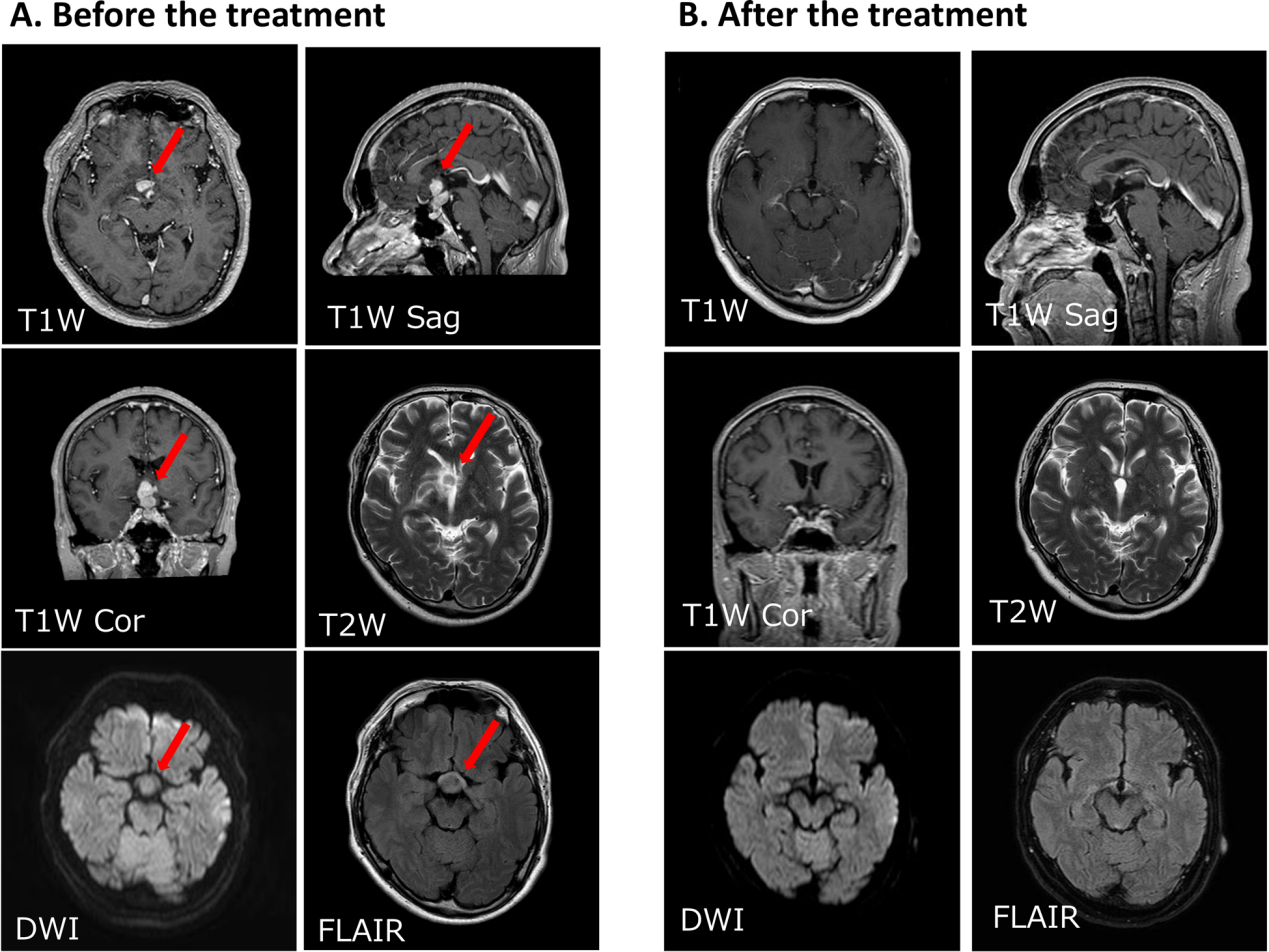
Imaging analysis before and after the treatment of the central nervous system lymphoma. a, b. Gadolinium-enhanced magnetic resonance imaging (MRI) before and after the initial treatment of the patient’s central nervous system lymphoma. A 16 × 12 mm lobulated tumor mass in the hypothalamus that extends to the pituitary stalk and the right temporal lobe was detected as a low intensity mass in T2-weighted imaging, a faint high intensity mass in diffusion-weighted imaging, and fluid attenuated inversion recovery imaging (a). The mass was no longer detected after the treatment (b). T1, T1-weighted imaging; T2, T2-weighted imaging; DWI, diffusion-weighted imaging; FLAIR, fluid attenuated inversion recovery imaging; Sag, sagittal plane; Cor, coronal plane
